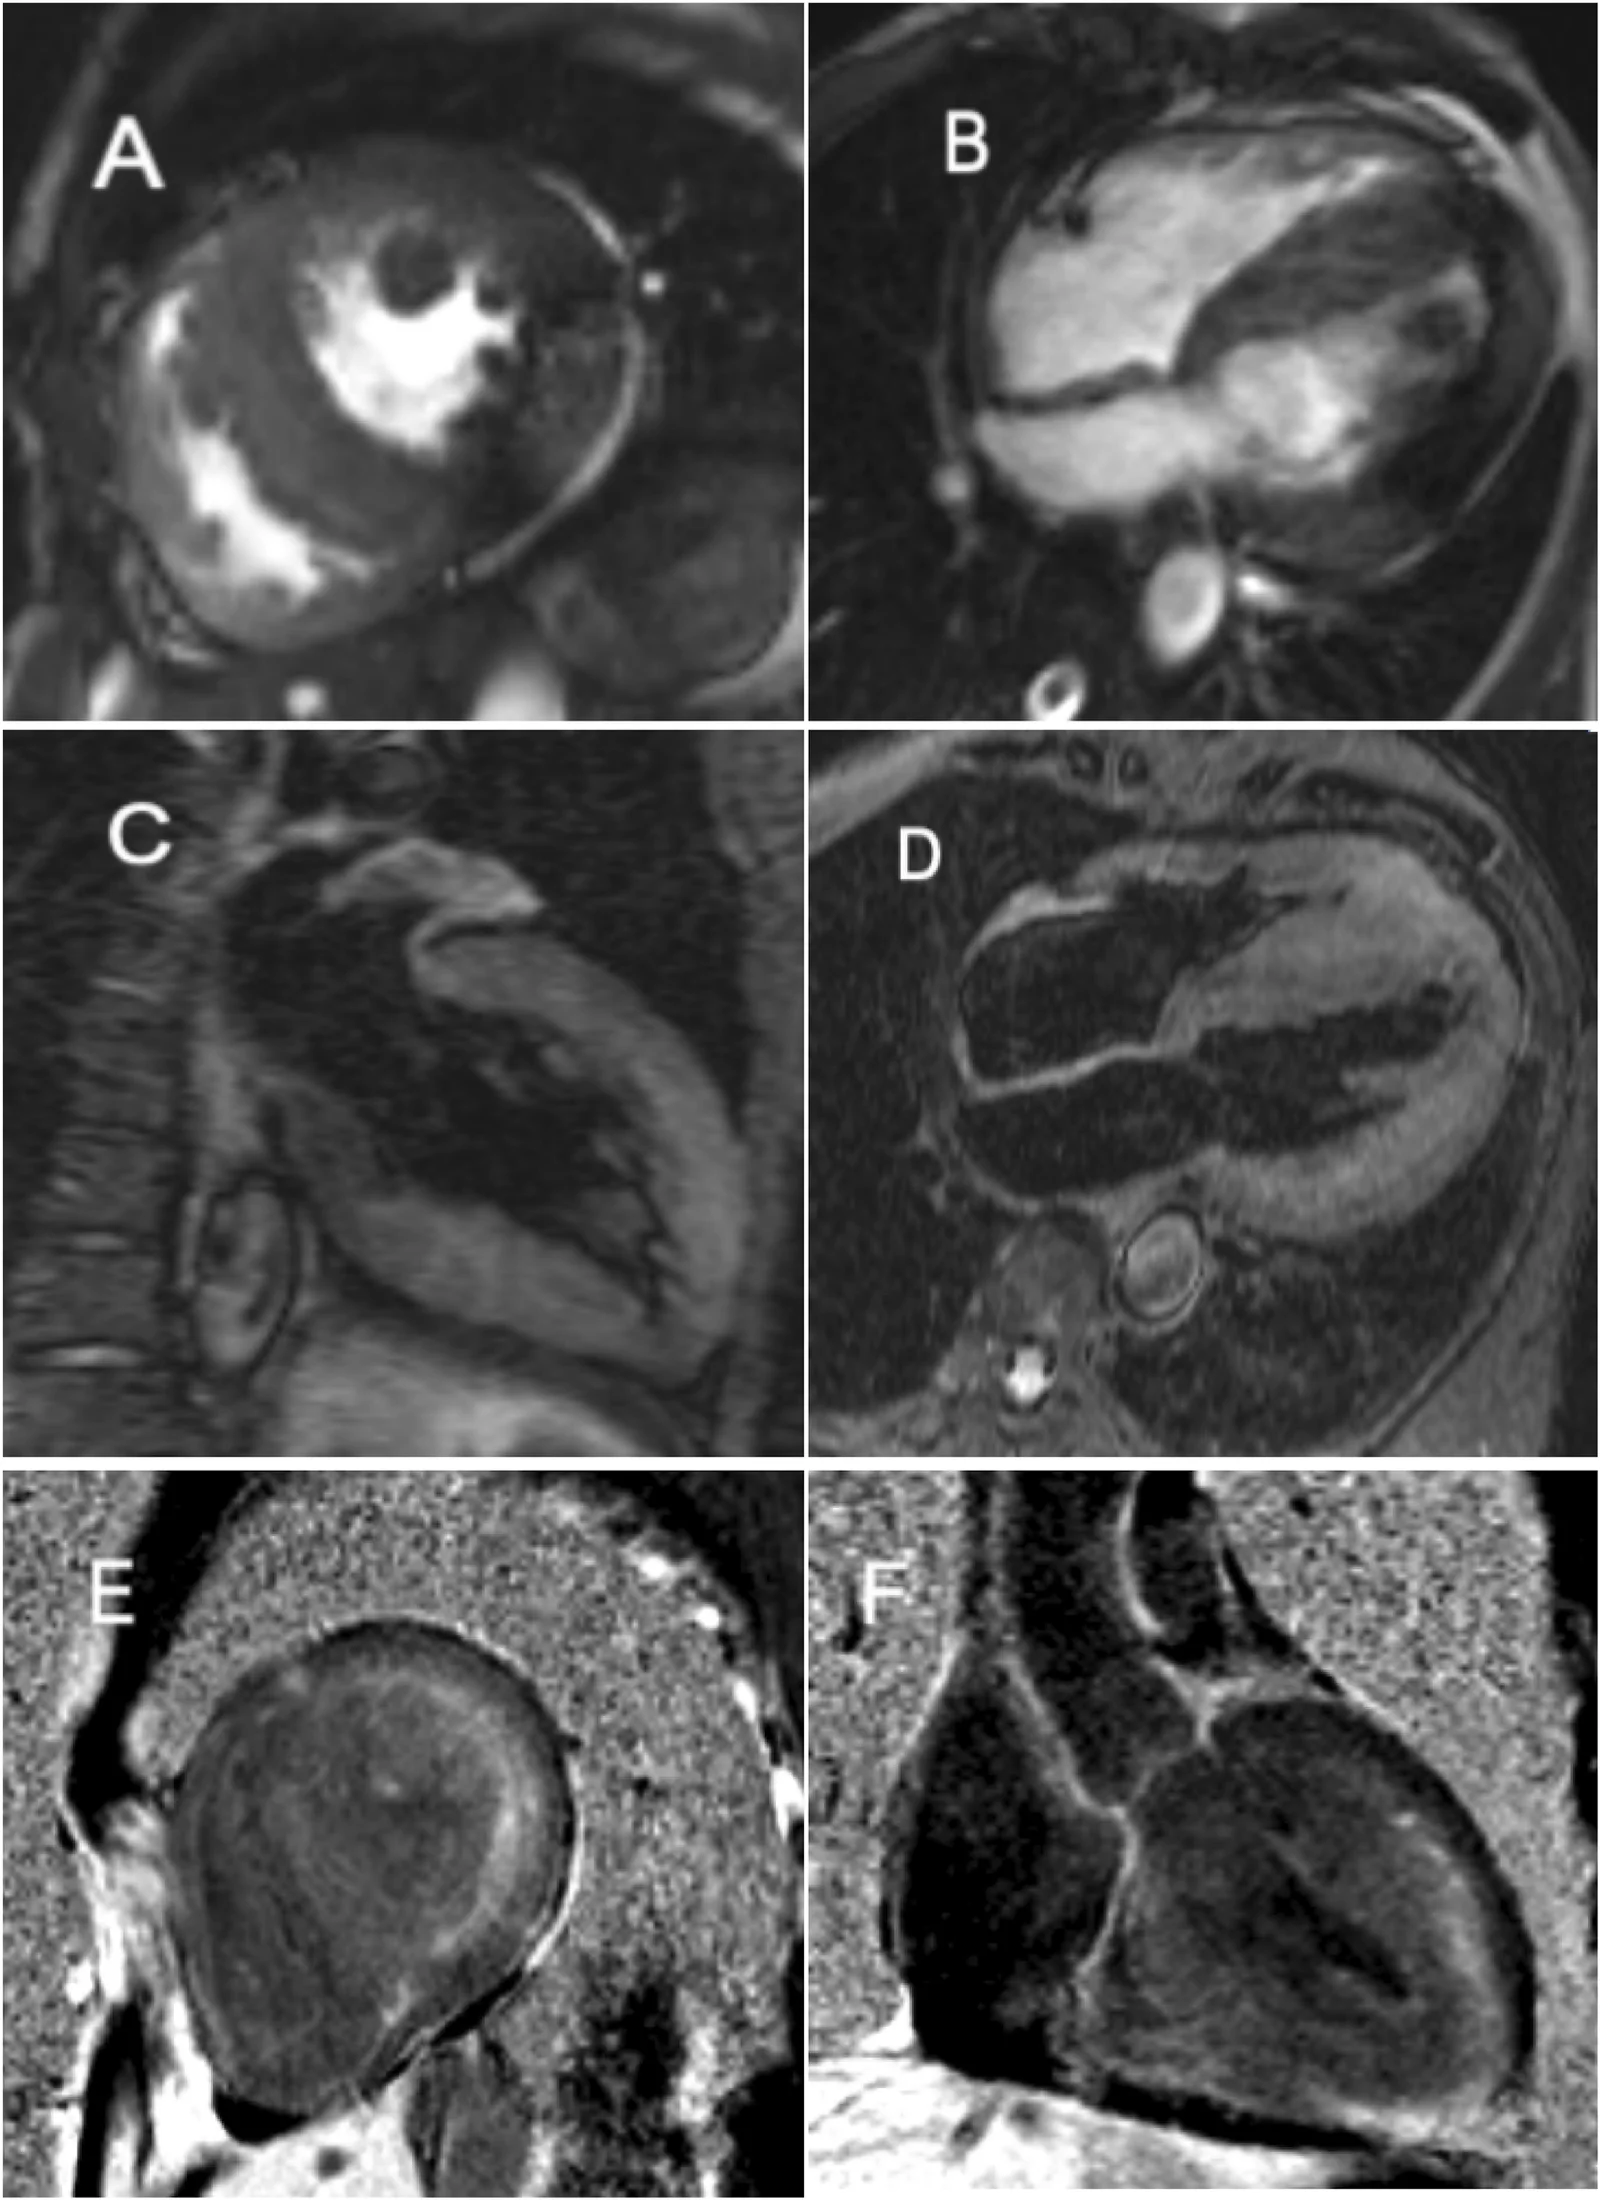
Cardiac magnetic resonance imaging (CMR) of the proband. Notes: Cardiac MRI (3.0 T) cine images (balanced steady-state free precession sequence) in the basal left ventricular short-axis (A) and four-chamber views (B) demonstrate marked asymmetric left ventricular hypertrophy (maximal wall thickness: 21 mm in the septum and 19 mm in the lateral wall). T2-weighted fat-suppressed images in the basal two-chamber (C) and four-chamber views (D) show myocardial edema. Late gadolinium enhancement (LGE) images in the basal short-axis (E) and four-chamber views (F) demonstrate extensive subendocardial fibrosis of the left ventricular wall, with a zebra sign in the interventricular septum.
